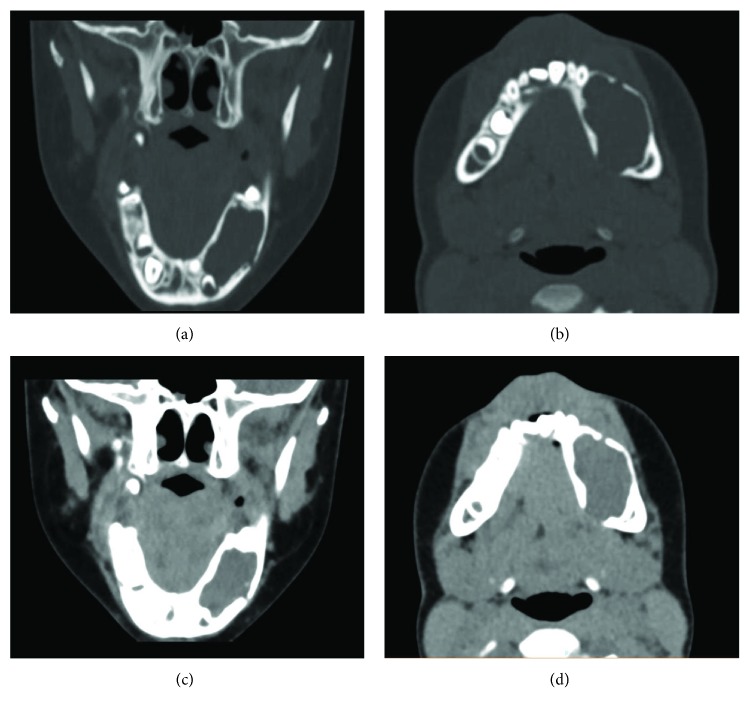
(a–d) Computed tomogram demonstrates an expansile mass to the left mandible with small buccal and lingual perforation of the cortices.
